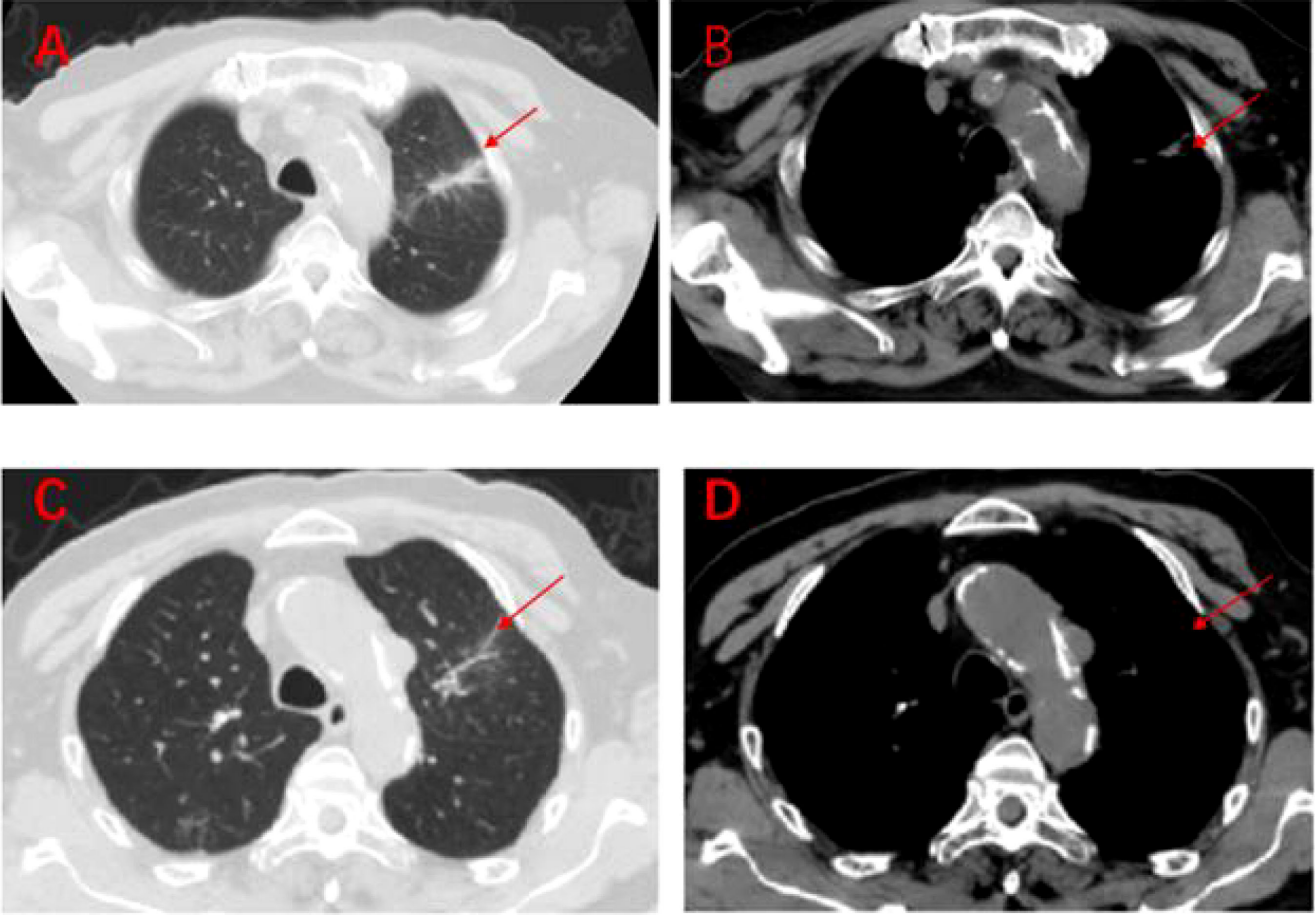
Regular imaging at follow-up, tumour lesions continue to shrink, efficacy maintained at PR (A–D). (A, B): On 7 March 2020, a lung CT scan revealed almost no tumour lesions. (C, D): On 3 October 2020, a lung CT scan revealed almost no tumour lesions.
